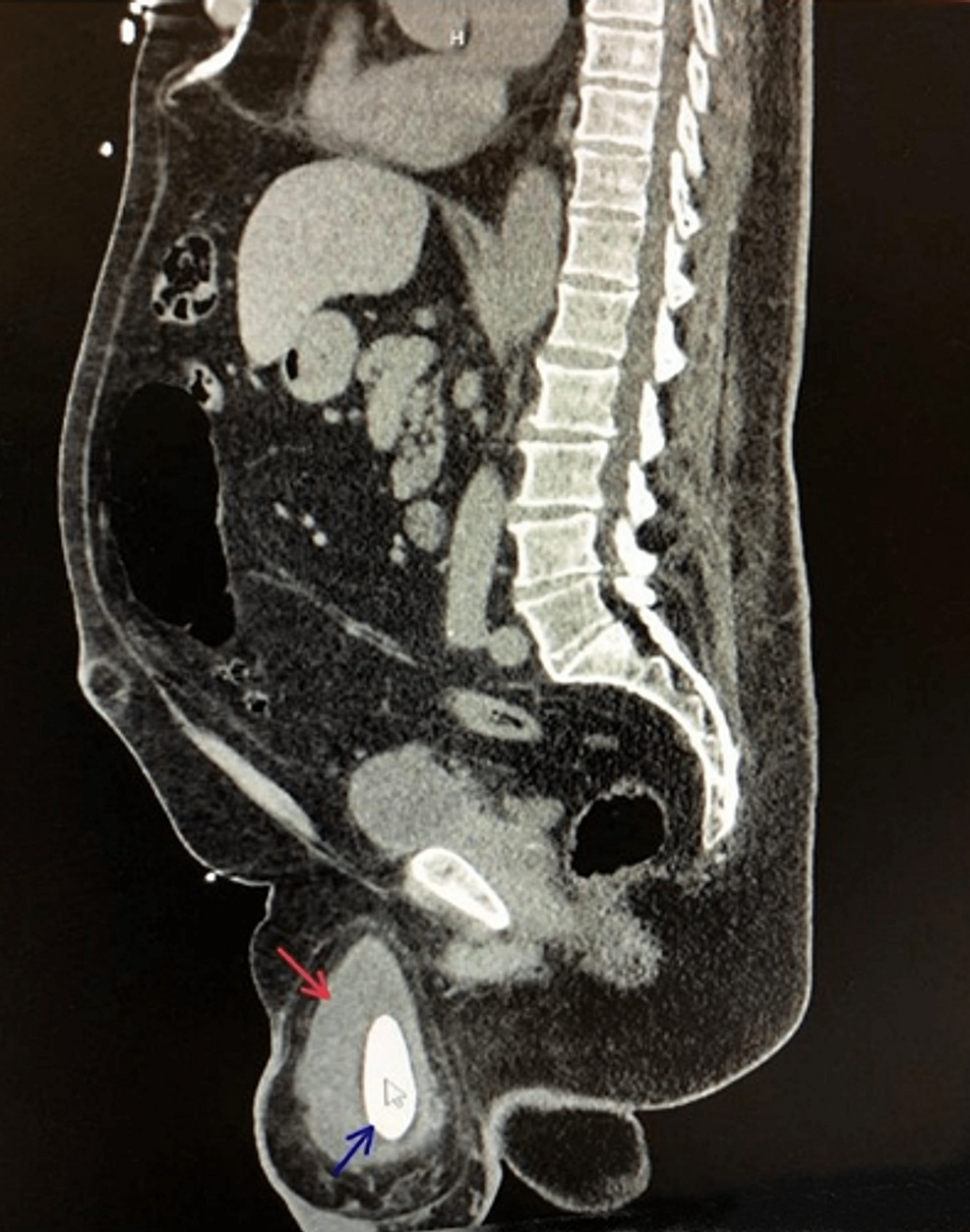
A sagittal CT scan showing a pantaloon urinary bladder herniation (red arrow) with a calculus (blue arrow).
